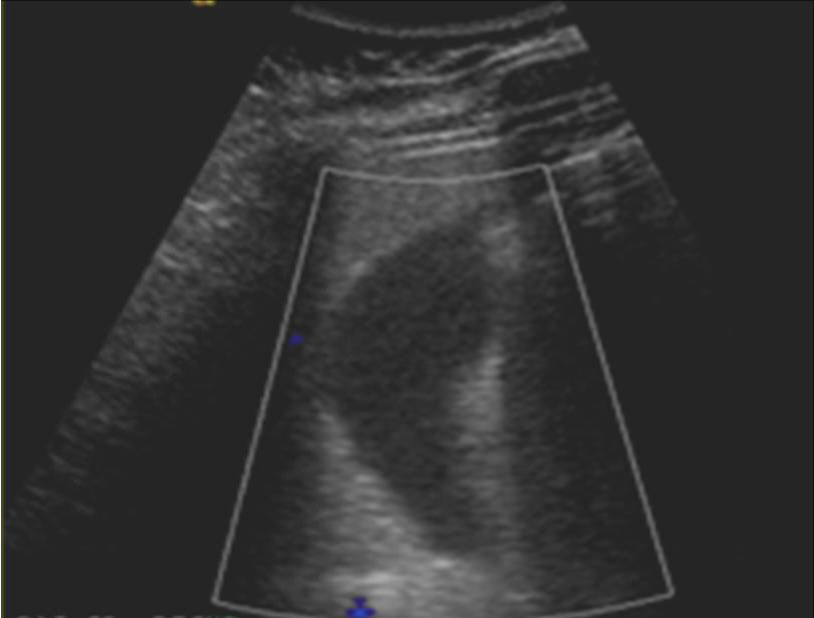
Abdominal ultrasonography showed no evidence for gallstones; common bile duct was not dilated and measured 0.5 cm.
